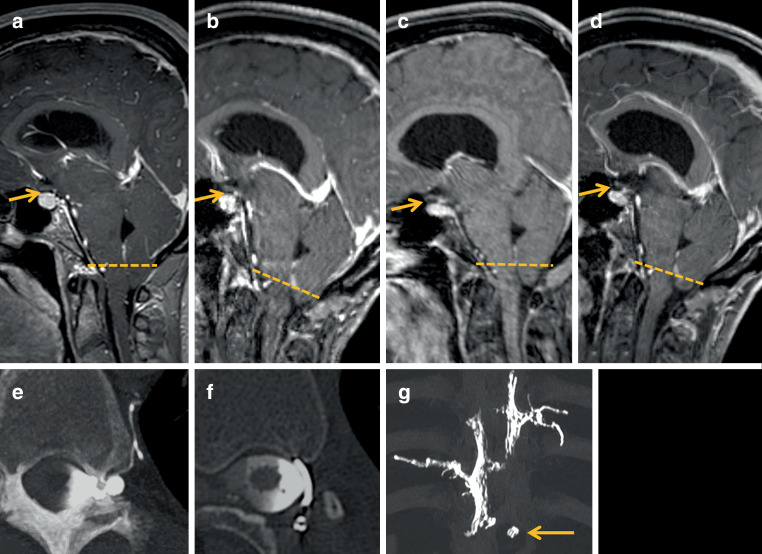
a Sagittal MRI from 2017 after 2 epidural blood patches (EBPs). Distinctive MRI signs with a Bern SIH score of 8 (pronounced pachymeninges and engorgement of sinuses are not shown), predominantly with brain sagging, abolished suprasellar distance (*arrow*) and severe tonsillar herniation (*dashed*
